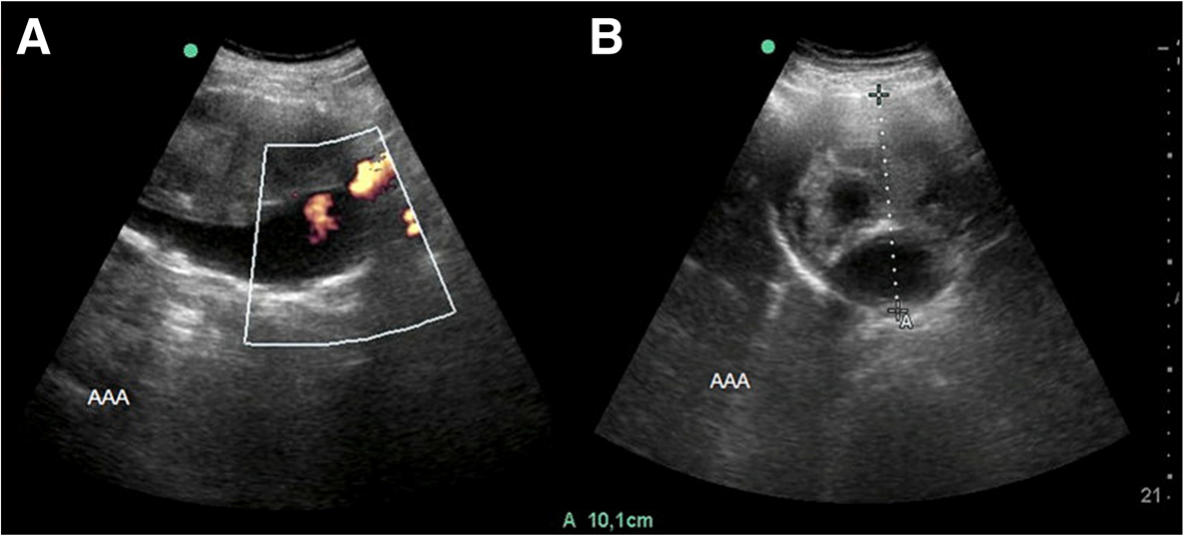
a, b. Ultrasonographic study in a 65 year old male patient with an Abdominal Aortic Aneurysm (AAA) (A), with a maximum diameter of 10.1 cm (cm) determined by this imaging study. *Permission to reproduce granted by Permanyer Hinojosa CA, Bermudez-Serrato K, Anaya-Ayala JE,* et al. *Proactive measurements in the search of aortic aneurysms have an impact in the prevalence. Cir Cir. Article in press*
